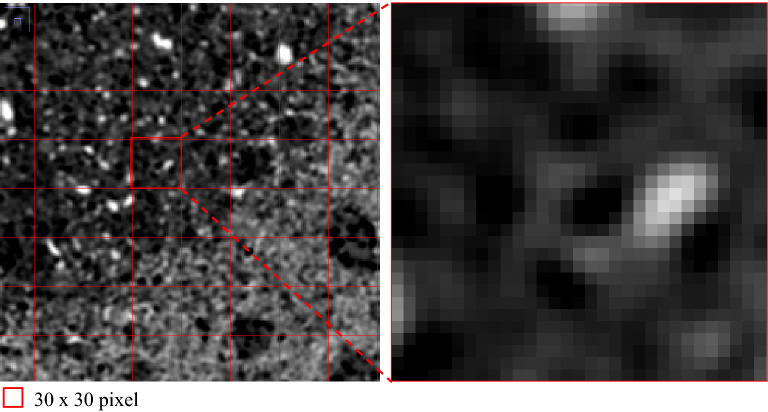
A typical cross-sectional CT image of a porous sample of sintered lunar regolith simulant consisting of pixels with different gray intensity values.
